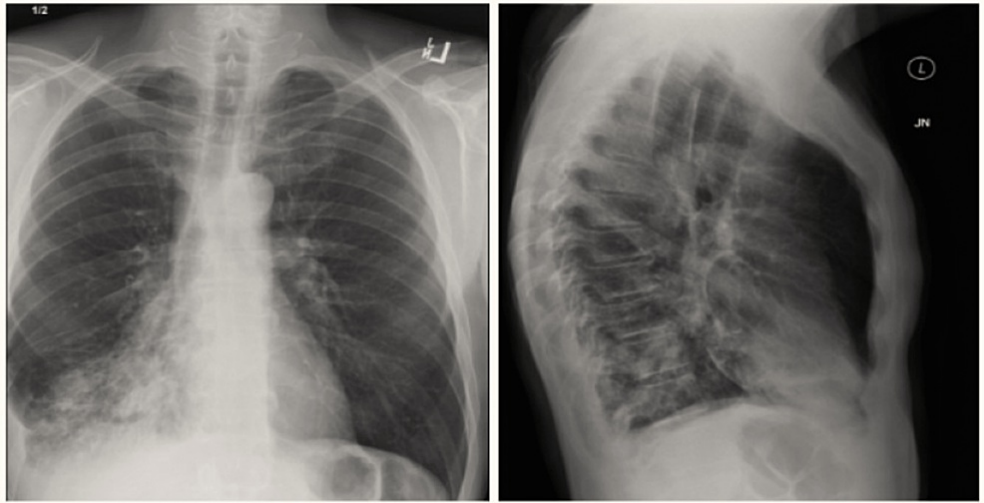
Chest X-ray showing right lower lobe consolidation
